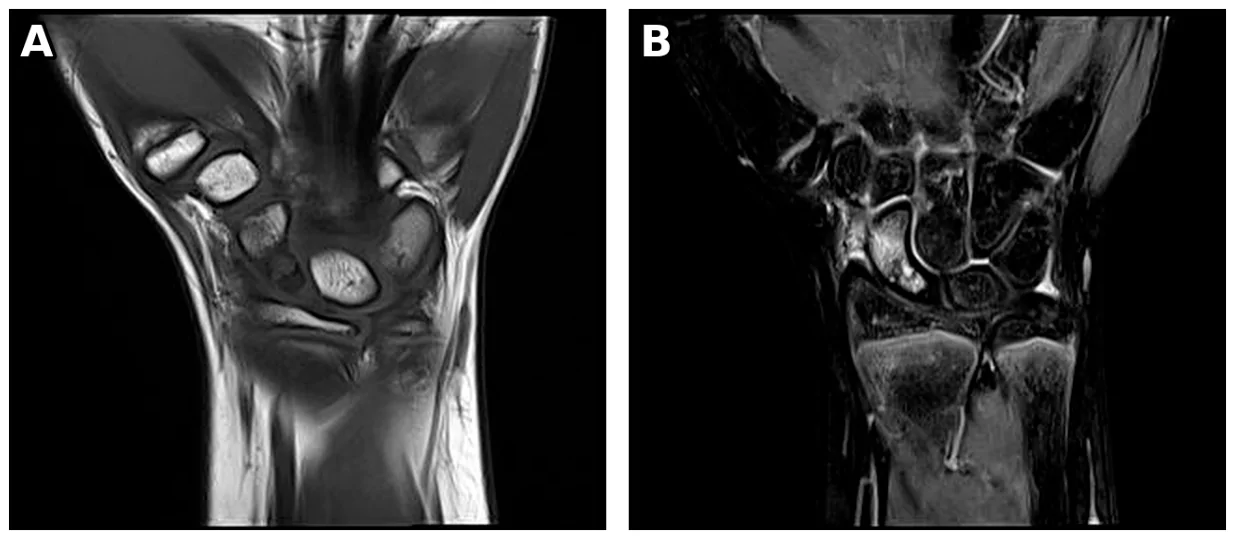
MRI assessment of scaphoid viability. (A) Coronal T1-weighted image showing preserved fatty marrow signal in both fragments. (B) Coronal fat-suppressed fluid-sensitive image showing increased signal at the nonunion interface. No MRI evidence of osteonecrosis was identified.
